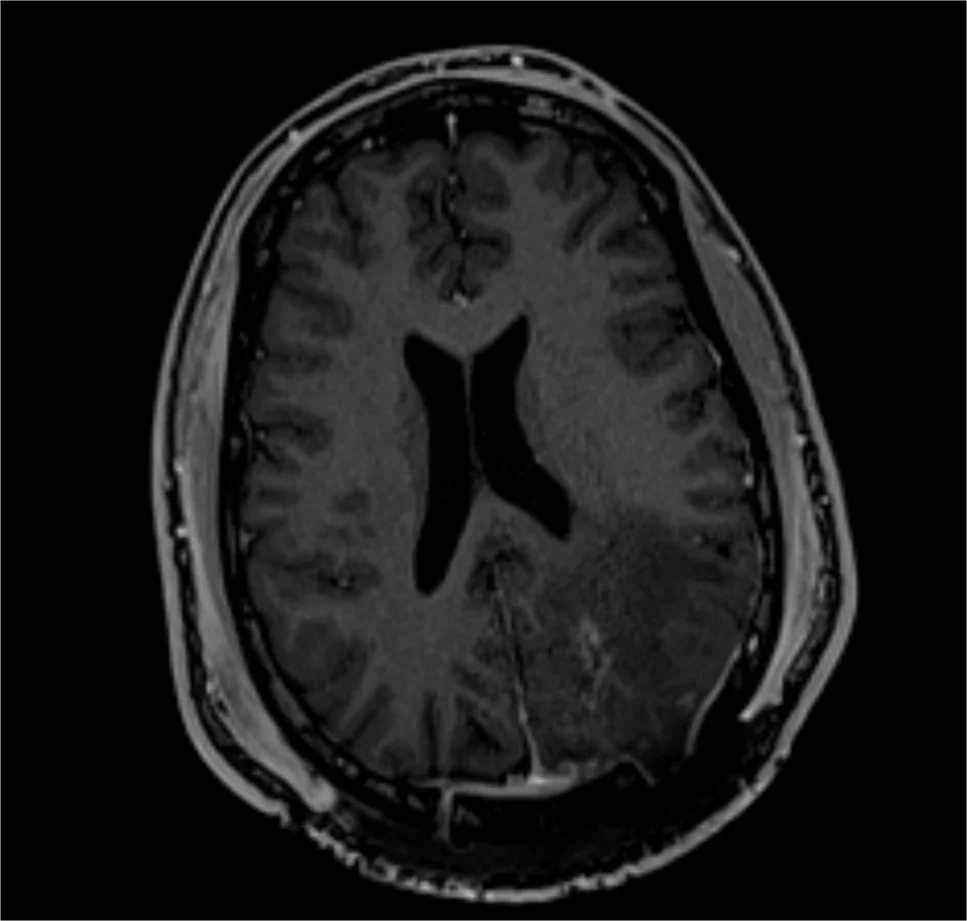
MRI brain T1 post-contrast sequence obtained after re-resection of the lesion, demonstrating gross-total resection
